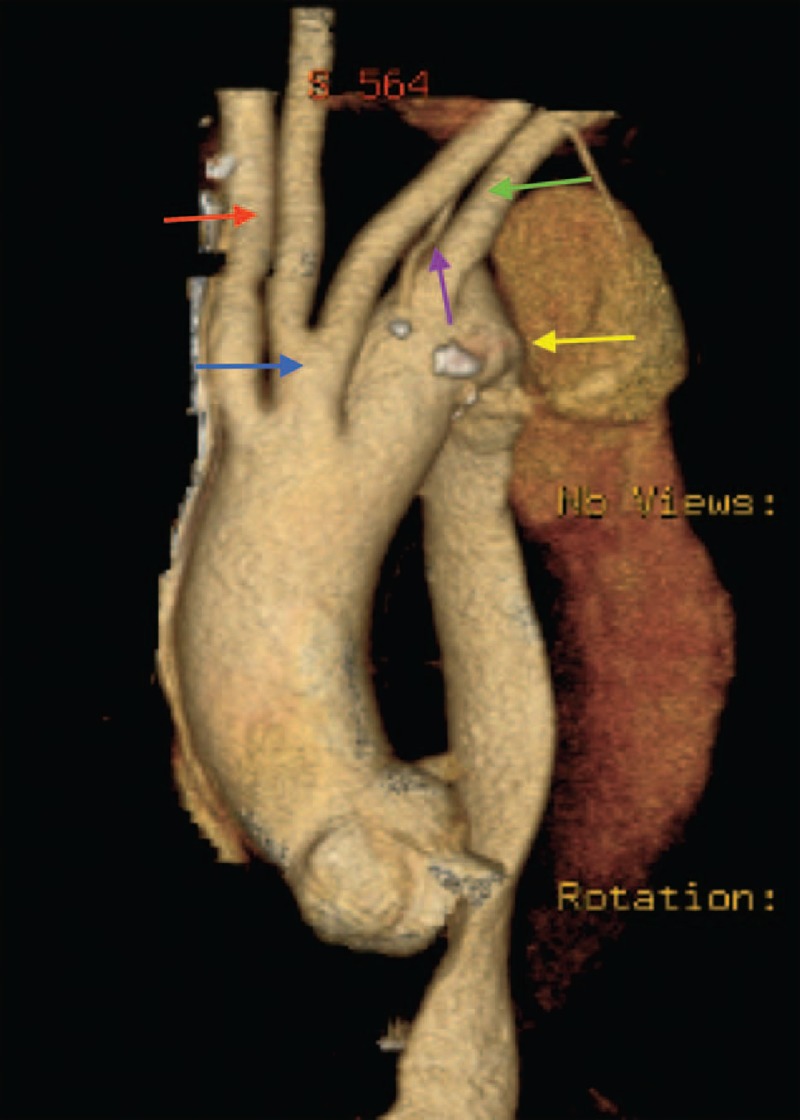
CT scan image (volume rendered). LSCVS and Stanford B aortic dissection. (Red arrow, right subclavian artery; blue arrow, common trunk of the carotid arteries; purple arrow, vertebral artery with direct origin of the arch; green arrow, left subclavian artery; yellow arrow, dissection.) CT = computed tomography, LSCVS = left aortic arch with right subclavian artery, common origin of carotid arteries, vertebral and left subclavian arteries.
